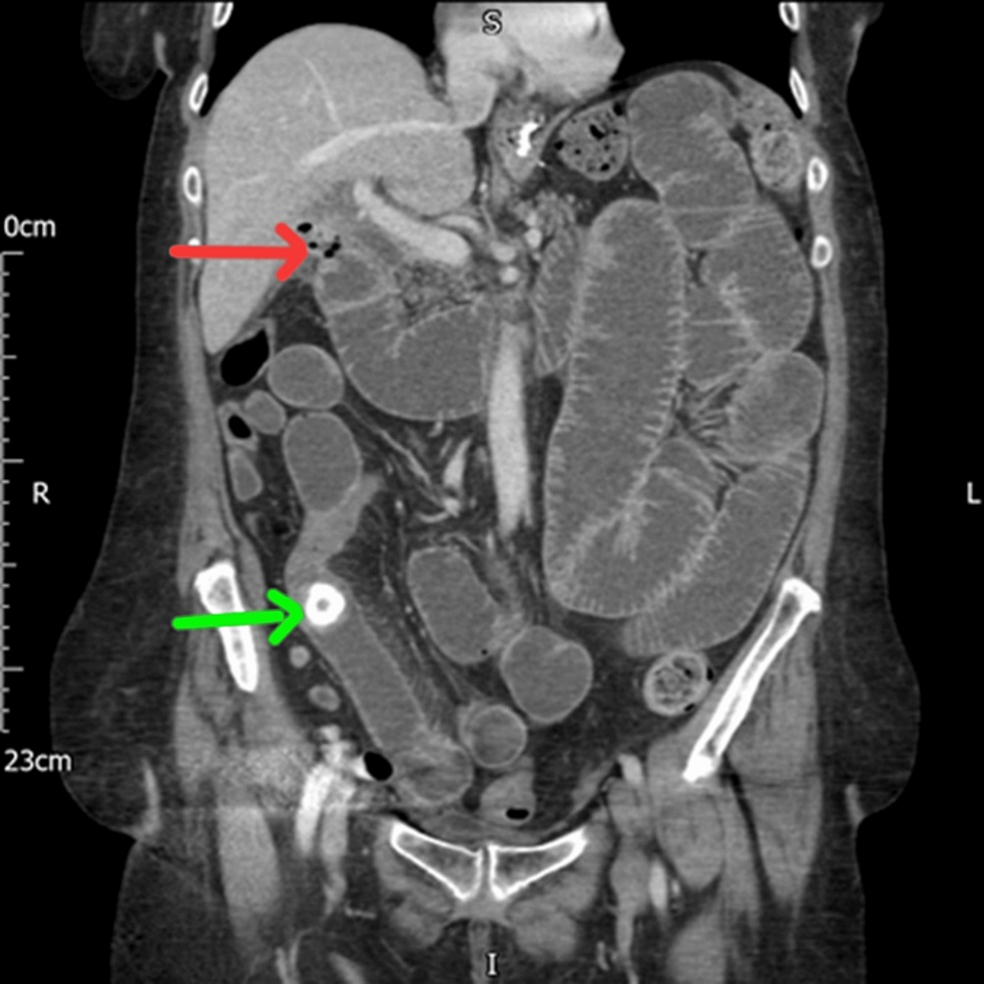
CT of the abdomen coronal view showing stone in the terminal ileum (the green arrow) and cholecystoduodenal fistula (the red arrow) CT: computed tomography
